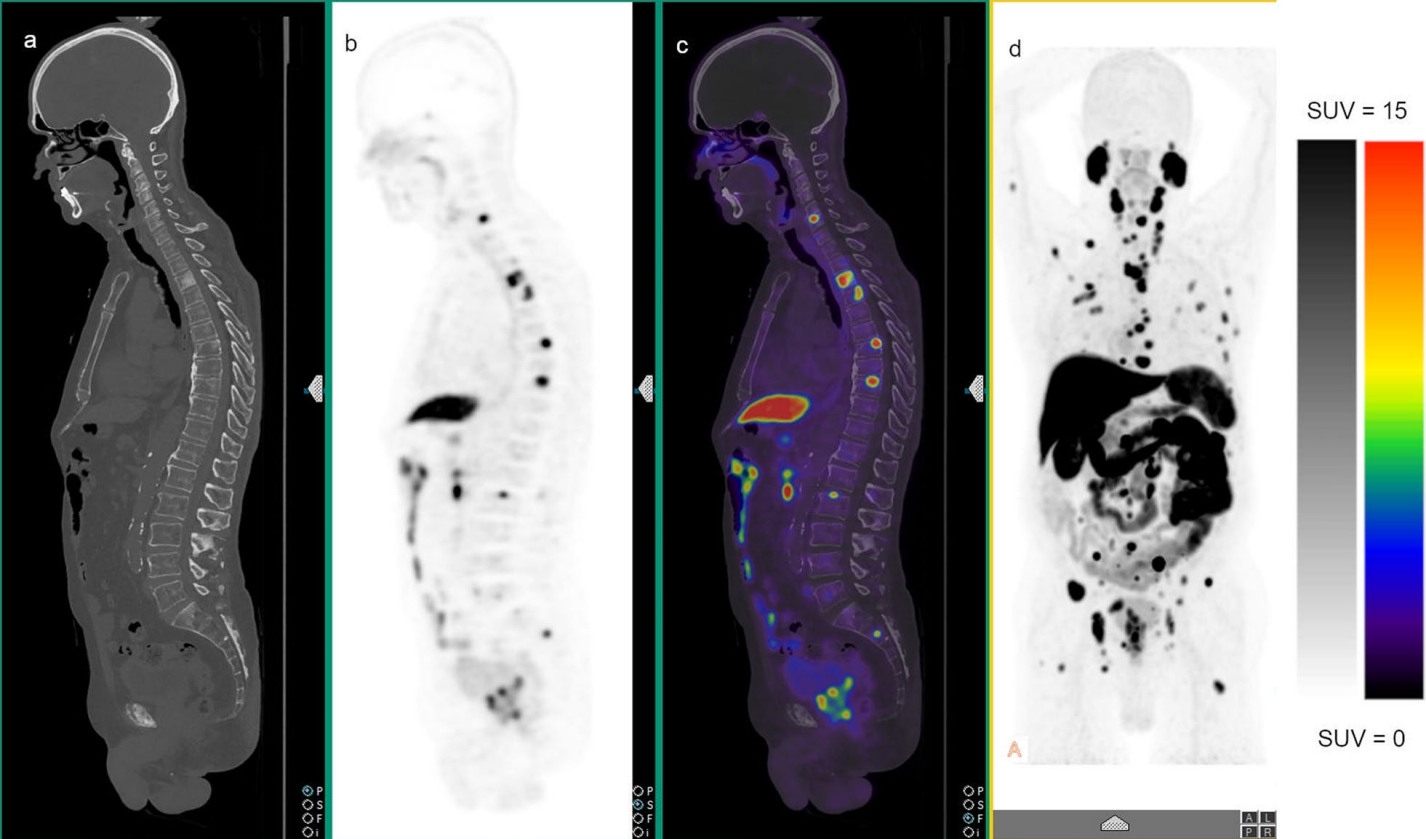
An 88-year-old male with high-risk prostate cancer (ISUP grade 4; PSA 142 ng/mL) underwent [^18^F]PSMA-1007 PET/CT demonstrating intensely F-PSMA avid primary tumour (SUVmax 17.8) and multifocal F-PSMA avid distant metastatic disease. a Sagittal non-contrast CT image on bone window, b sagittal PET attenuation correction image and c sagittal fused PET/CT image from the vertex to pelvis. d Maximum intensity projection (MIP) image
